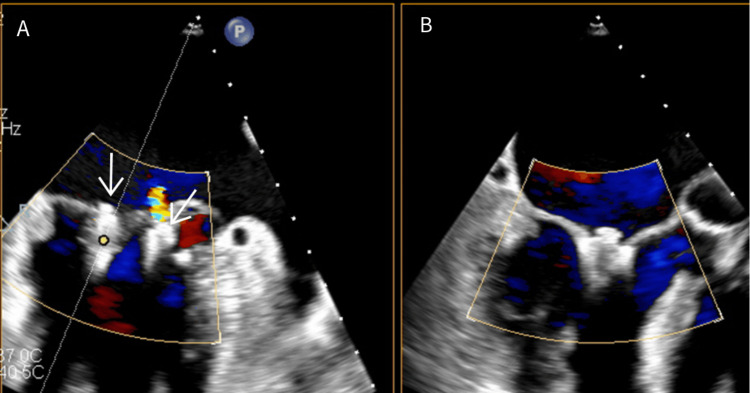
Transesophageal echocardiogram (A) Two mitral valve edge-to-edge devices inserted centrally between A2/P2 and laterally (white arrows). (B) Demonstration of only mild residual mitral regurgitation.
